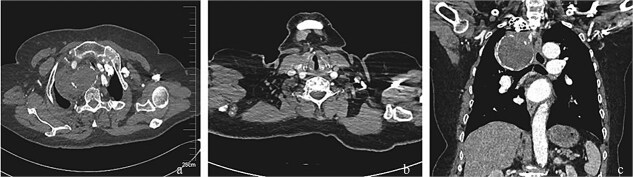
Post-interventional imaging surveillance at 4-month follow-up. (a) Axial CTA demonstrates complete thrombosis of the aneurysm sac following successful flow isolation, with volume reduction compared to pretreatment dimensions. (b) Sagittal reconstruction confirms absence of right vertebral artery opacification, consistent with deliberate ostial exclusion during the index procedure. (c) Coronal volume-rendered technique delineates organized thrombus within the collapsed aneurysm cavity, patency of the stent-graft lumen, and preserved distal subclavian artery perfusion through collateral channels. The radiopaque coil mass at the outflow tract confirms successful embolization endpoint achievement.
